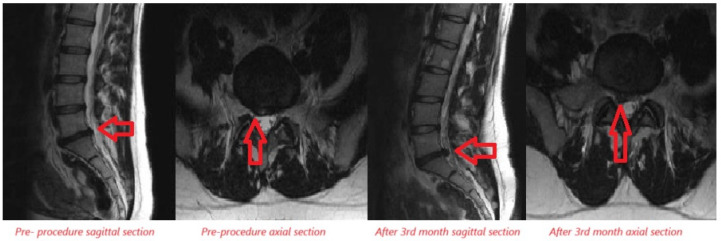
Examination of disc size increase after ozone + PRP combine therapy in lumbar MRI sagittal and axial sections. MRI, magnetic resonance imaging; PRP, platelet-rich plasma.
